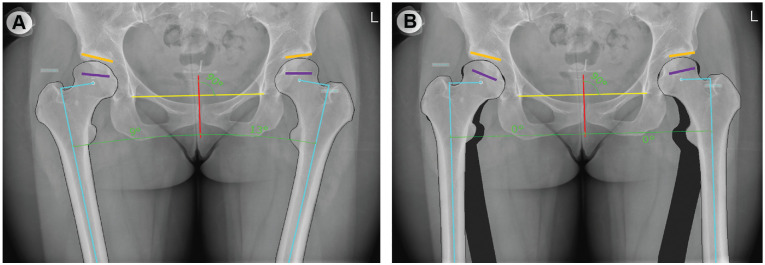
A pelvic anteroposterior radiograph demonstrating (A) the initial position of the hips as taken in the radiology department and (B) after correction of the hip adduction/abduction angle. The hip adduction/abduction angle was defined as the angle between a vertical line (red line) to the tangent to the pelvic teardrops (yellow line) and the femoral shaft axis (light blue line). The acquired radiograph demonstrated 13° of adduction on the left side and 9° of adduction on the right side. The FEAR index was defined as the angle between the physeal scar (purple line) and the sclerosis of the acetabular sourcil (orange line). The uncorrected FEAR index demonstrated in (A) is positive (lateral opening angle), whereas the corrected FEAR index, demonstrated in (B) is negative (lateral closing angle). FEAR, femoroepiphyseal acetabular roof.
